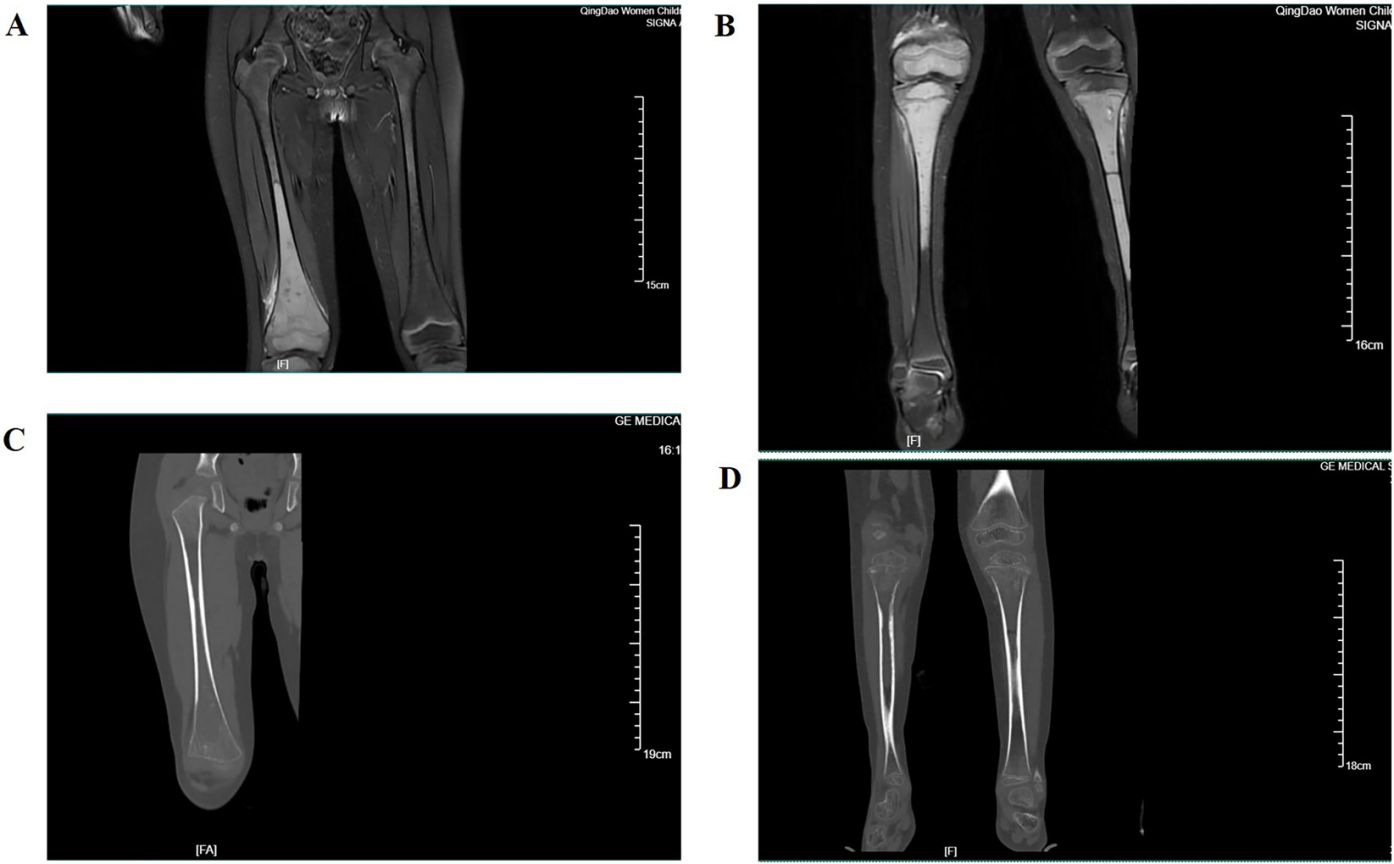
Findings of MRI and CT scan revealed pathological fracture of right distal femur and bilateral proximal tibiae, and indicated the possibility of metabolic bone disease or tumor-like lesion. A. MRI scan of bilateral femurs. B. MRI scan of bilateral tibiae and fibulae. C. CT scan of right femur. D. CT scan of bilateral tibiae and fibulae.
